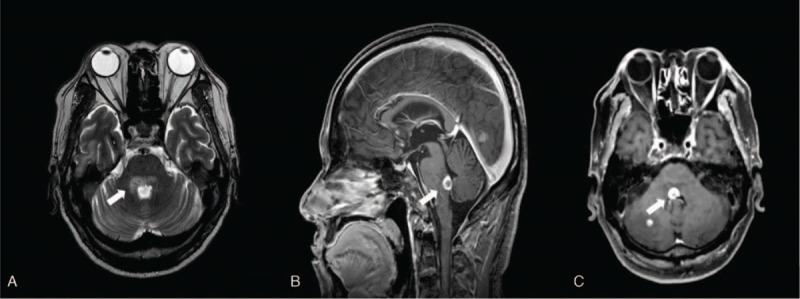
(A) Axial T2-weighted magnetic resonance imaging (MRI) image of the patient showing hyperintense lesion at the right tegmental pons (arrow). (B, C) Multiplanar reconstruction MRI saggital and axial images showing a high-signal-intensity lesion on the right pontine tegmentum (arrow).
